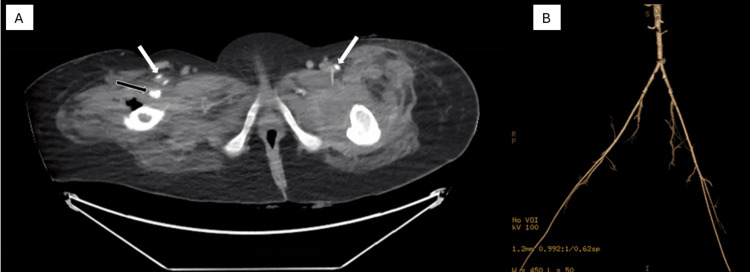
Preoperative computed tomography angiography (CTA) images showing patent major arteries without arterial abnormality in the inguinal regions A) Axial CTA showing the bilateral superficial femoral arteries (white arrows) and the right profunda femoris artery (black arrow). B) Angiographic three-dimensional volume-rendered image showing arterial patency.
